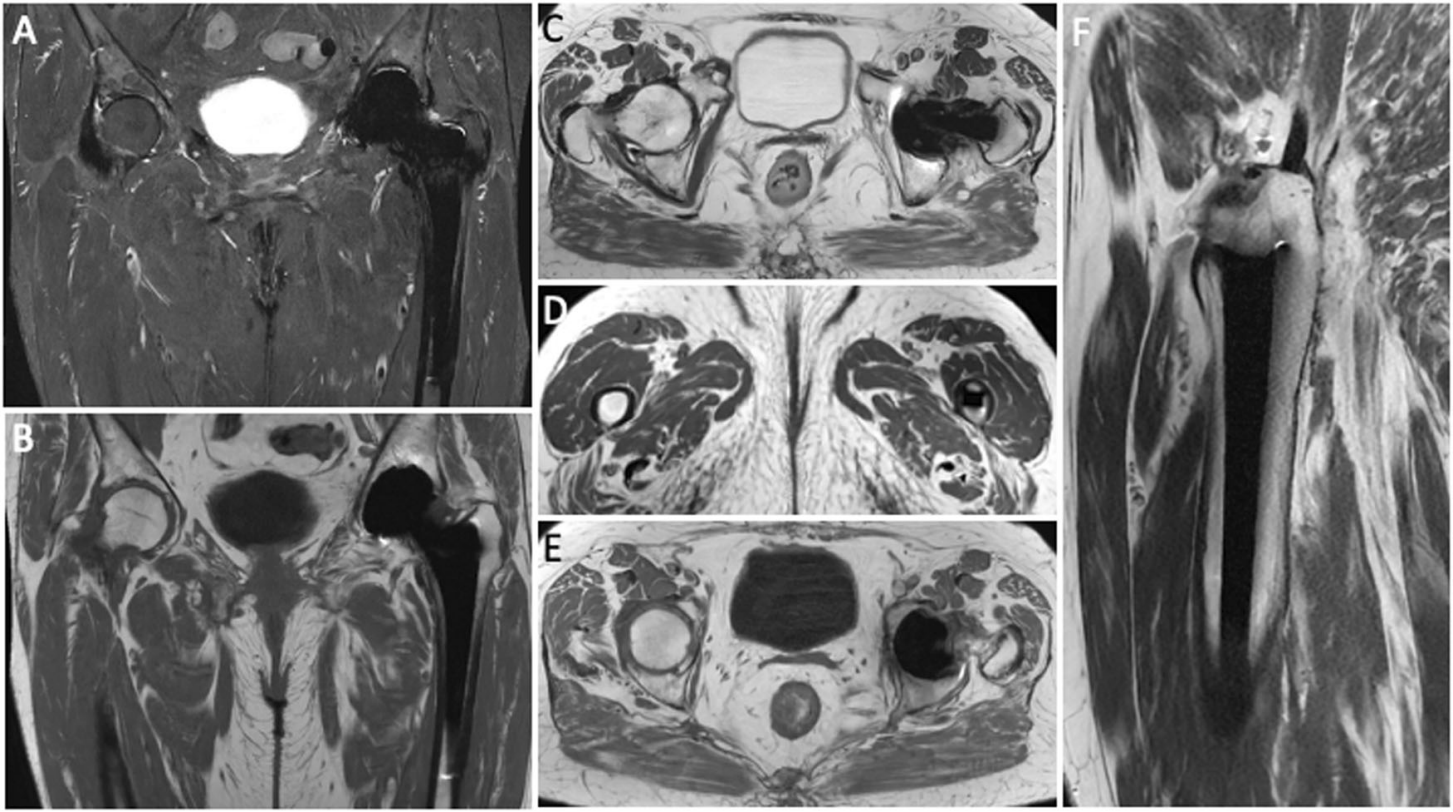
Pelvis MARS MRI of an asymptomatic 69-year-old female with normal left THA. Coronal STIR (A), coronal T1-weighted (B), axial T2-weighted (C and D), axial T1-weighted (E) and sagittal T2-weighted (F) images. No distortion or incomplete fat suppression is observed in these images. Periprosthetic bone is well-depicted without edema or osteolysis. MR images do not highlight other signs of painful THA like effusion, synovitis, collections, pericapsular edema, or masses. Further, no enlarged loco-regional lymph nodes are seen
